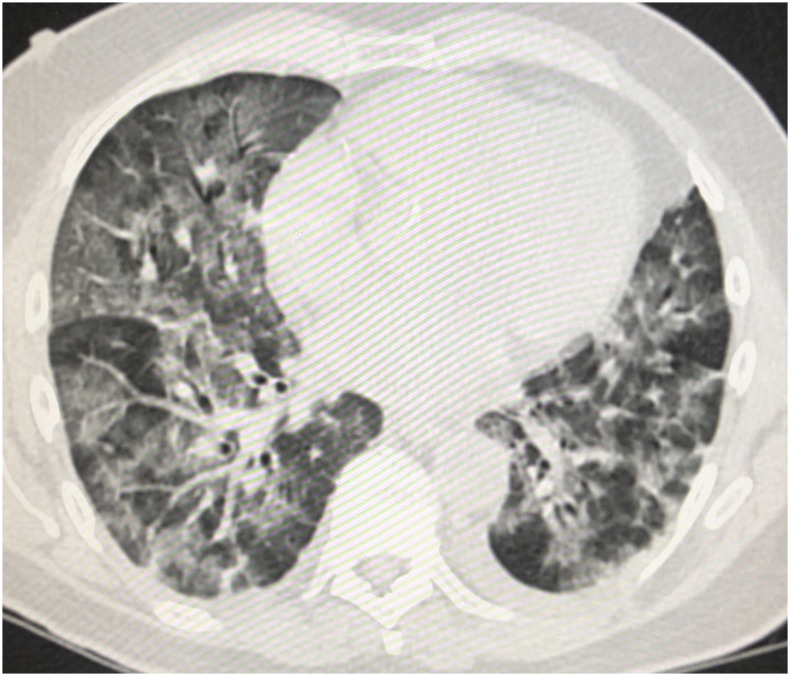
Computed tomography revealing bilateral infiltrates consistent with acute respiratory distress syndrome.
